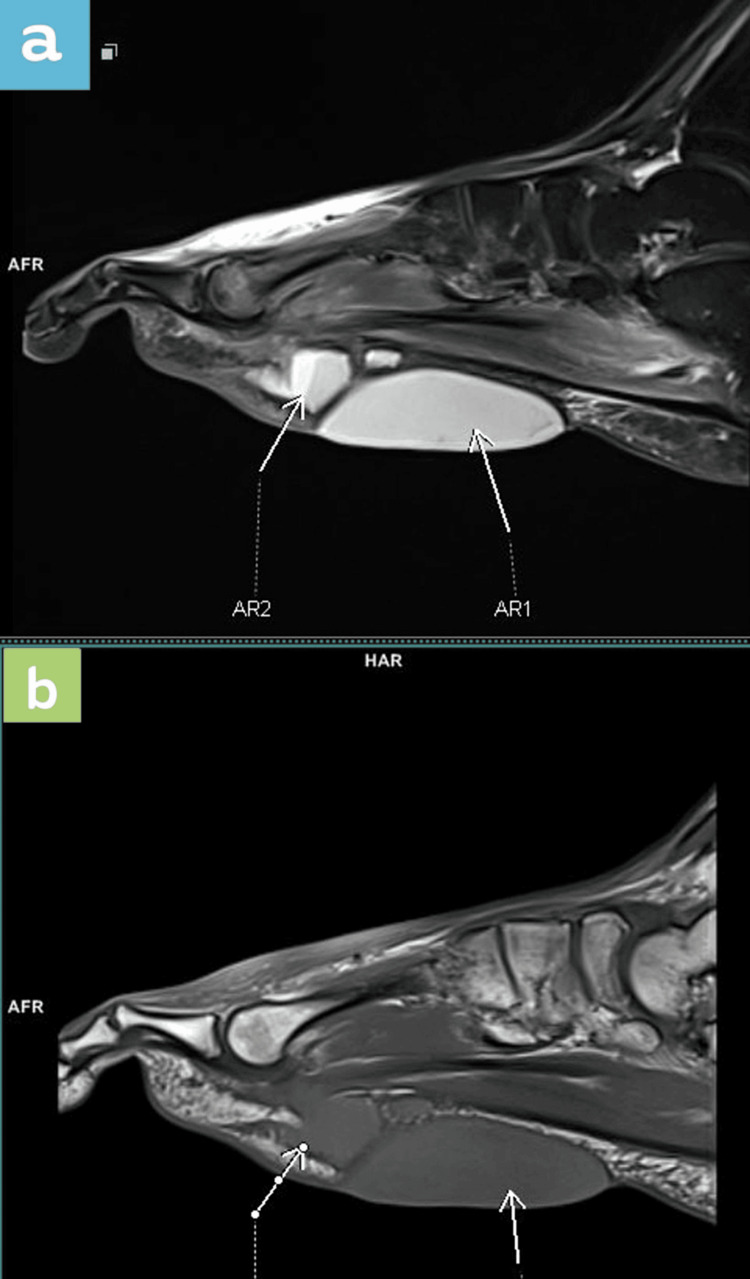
Sagittal MRI sequences showing both serous collections Top: a) proton density fat saturated (PDFS). Bottom: b) T1.
